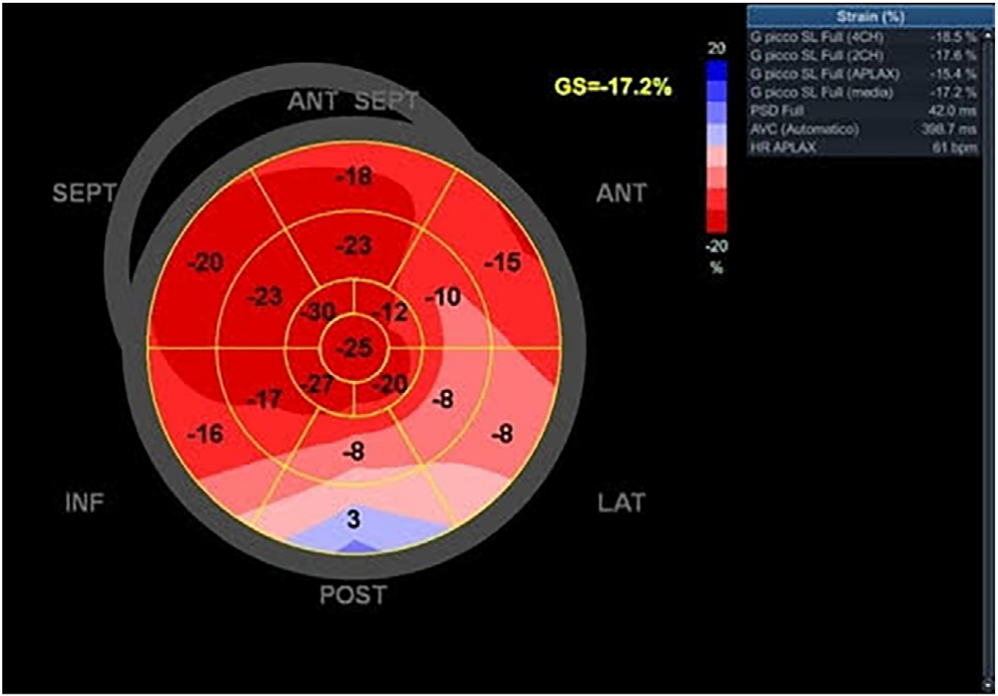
Global Longitudinal Strain (GLS) analysis performed using speckle tracking echocardiography; apical segments display preserved strain, suggestive of absence of myocardial involvement from cardiac amyloidosis, while posterior segments exhibit markedly reduced deformation.
